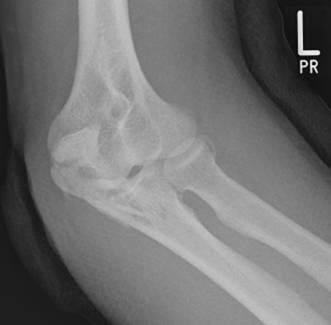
Sometimes an intermediate fragment is not detectable in conventional biplanar X-rays.
